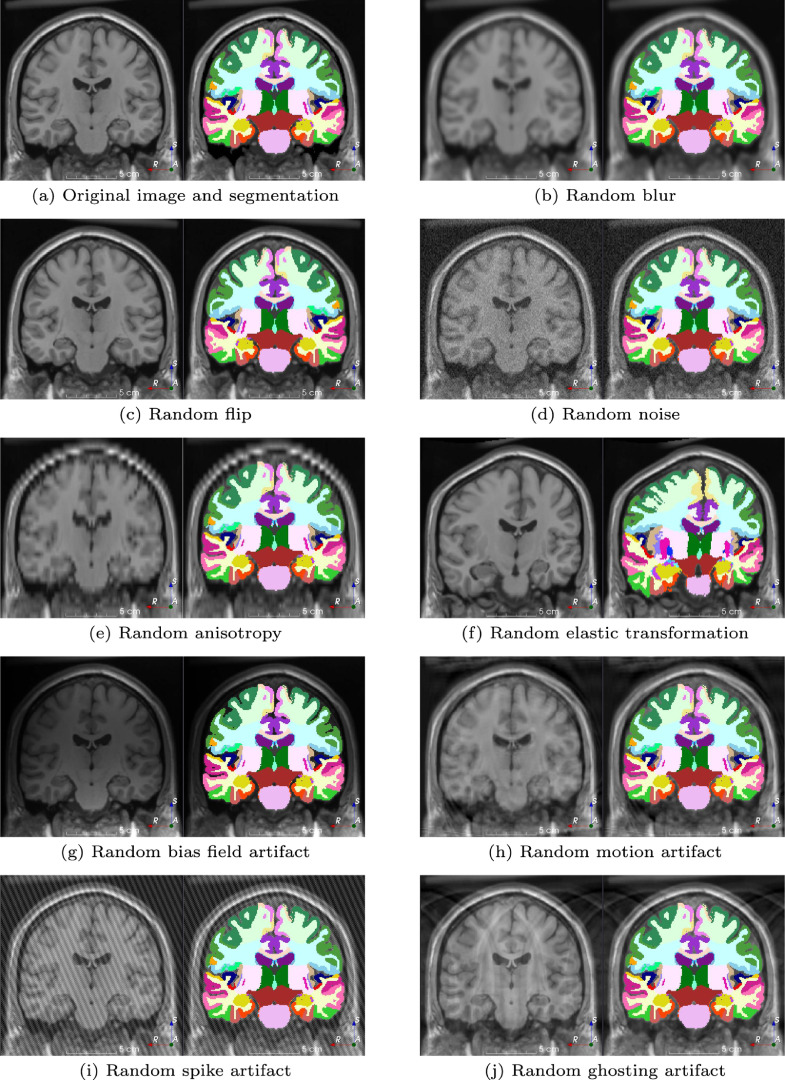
A selection of data augmentation techniques available in TorchIO v0.18.0. Each example is presented as a pair of images composed of the transformed image and a corresponding transformed label map. Note that all screenshots are from a 2D coronal slice of the transformed 3D images. The MRI corresponds to the Montreal Neurological Institute (MNI) Colin 27 average brain [49], which can be downloaded using torchio.datasets.Colin27. Label maps were generated using an automated brain parcellation algorithm [12].
